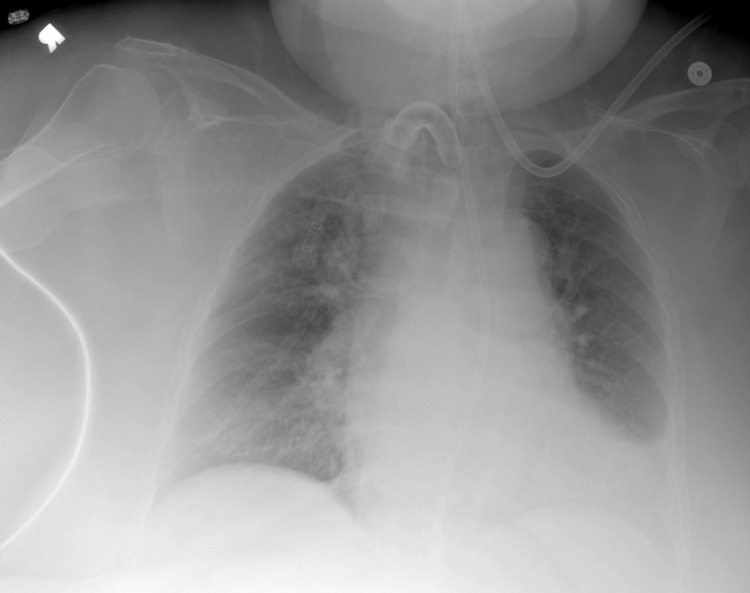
Chest X-ray obtained on the 23rd day of admission showing marked improvement of the right-sided pulmonary consolidation/edema
